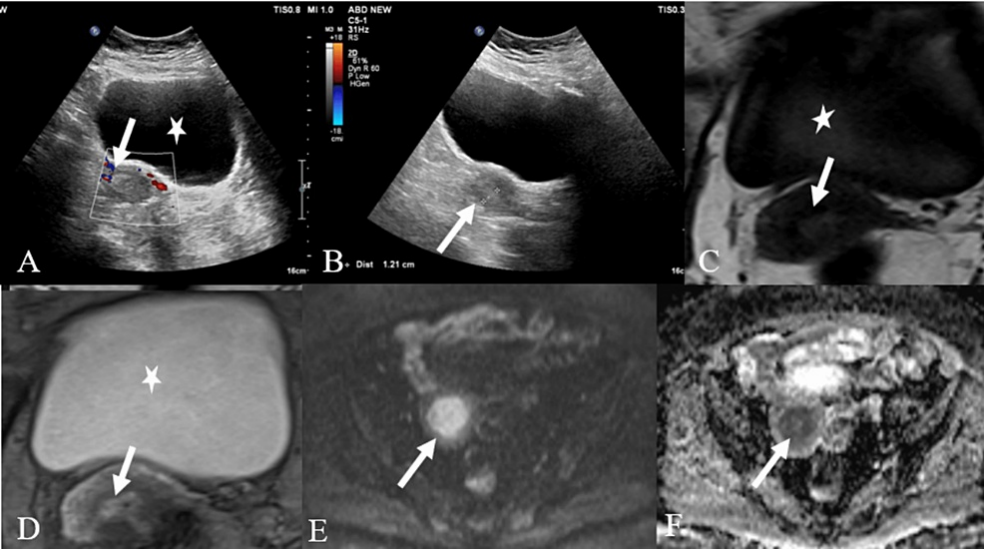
A case of endometrial carcinoma A: USG color Doppler image of the pelvis showing thickened and heterogenous endometrium (white arrow), demonstrating peripheral vascularity. B: USG of the pelvis showing thickened and heterogenous endometrium (white arrow). The uterus appears atrophied. The urinary bladder is denoted by a star. C and D: Sagittal T1 and T2 pelvis MR images showing thickened and heterogeneously hyperintense endometrium (white arrow). The urinary bladder is denoted by a star. E and F: Axial DWI of MRI of the pelvis and corresponding ADC images show the restriction of diffusion of thickened endometrium (white arrow), suggestive of endometrial malignancy. USG - ultrasonography; DWI - diffusion-weighted imaging; ADC - apparent diffusion coefficient
